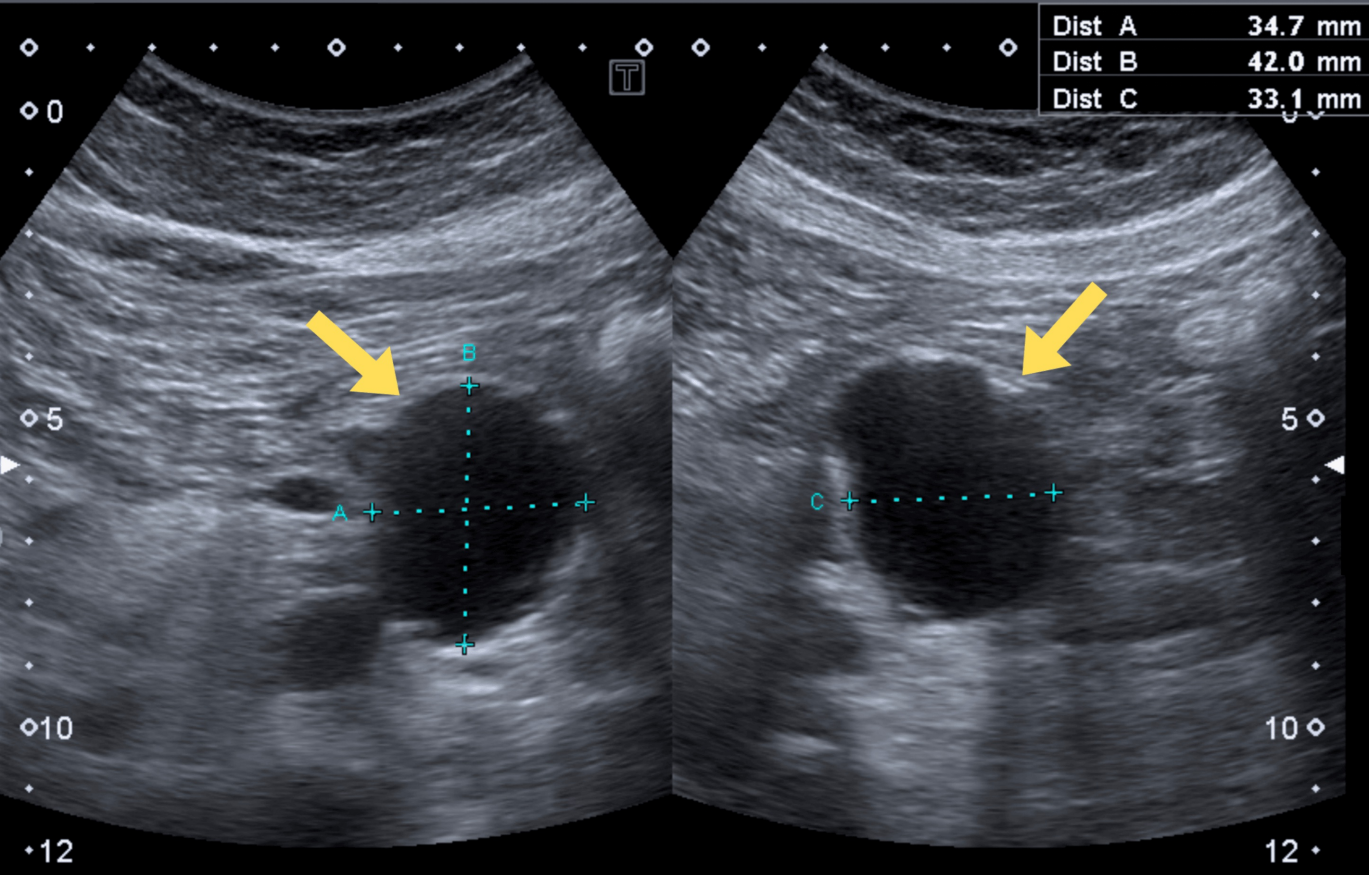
Ultrasound image showing an incidentally detected aneurysm. Yellow arrow indicates the superior mesenteric artery.
